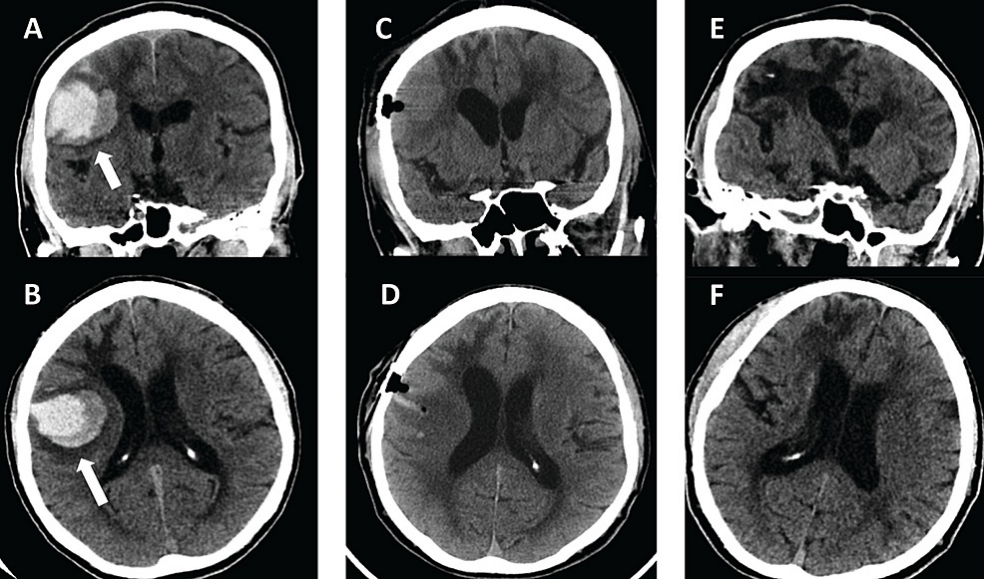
Case 2 - initial pre- and postprocedure and follow-up imaging. Preprocedure second admission non-contrast coronal (A) and axial (B) head computed tomography (CT) demonstrates a 42.5 mL right frontal hemorrhage (arrow). Postprocedure coronal (C) and axial (D) non-contrast CT demonstrated 86.8% evacuation with remaining hematoma measuring 5.6 mL. Coronal (E) and axial (F) follow-up imaging at one year showed the expected evolution of gliosis and encephalomalacia following hemorrhage.
